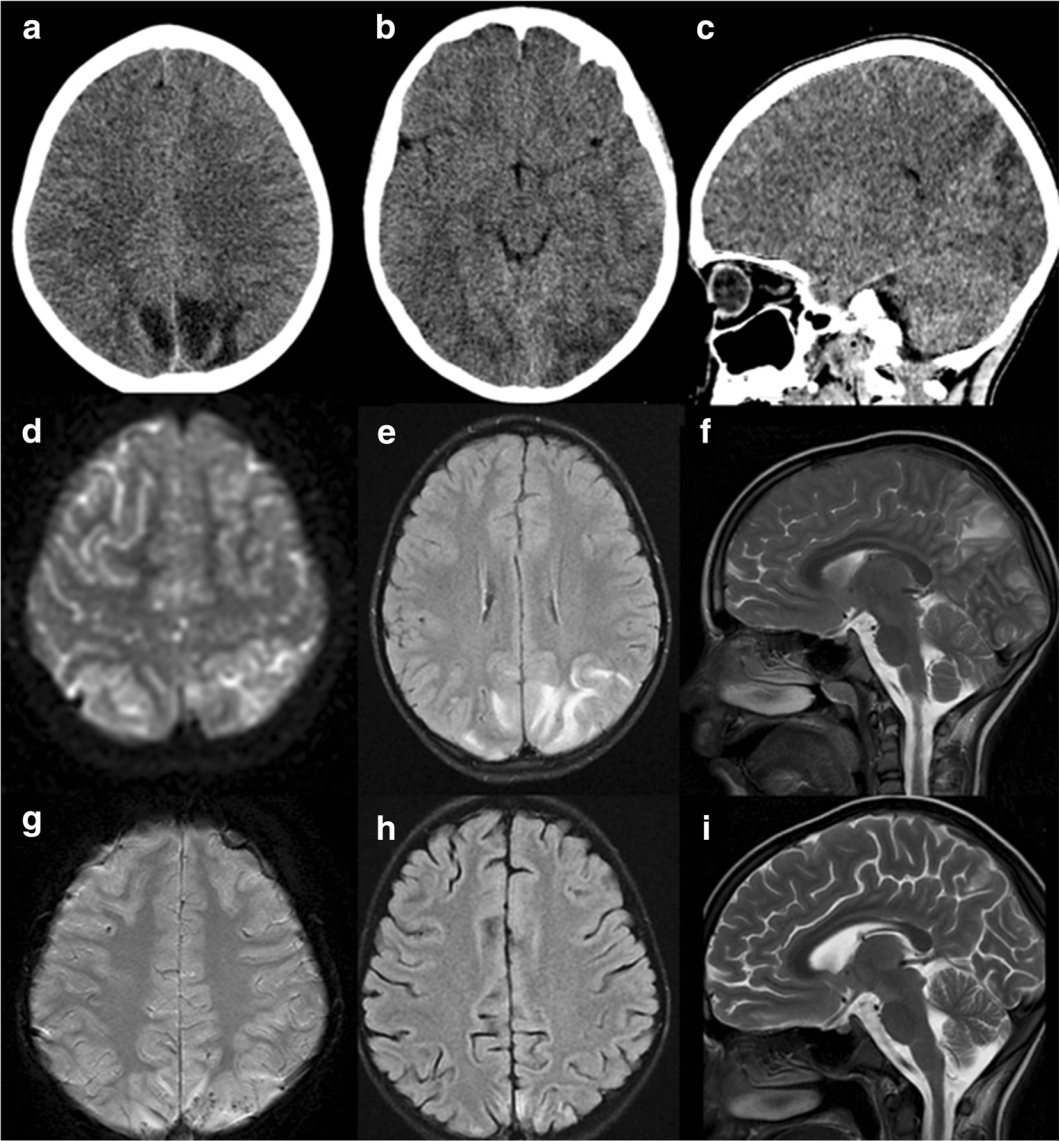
a–c Initial head CT axial plane (a and b) and sagittal multiplanar reconstruction (c): hypodense cortical-subcortical areas in the parietal lobes bilaterally and in the left occipital lobe—ischaemic changes with oedema. d–f Head magnetic resonance (MRI) in same day, axial diffusion-weighted image (DWI) (d): restriction of water diffusion, axial fluid-attenuated inversion recovery (FLAIR) image (e) and sagittal T2/weighted image (f): hyperintense signal in the parietal and occipital lobes—regions of cortical/subcortical oedema, correlating with PRES. g–i Control head MRI after 3 months, axial susceptibility weighted image (SWI) (g): small haemorrhagic regions, axial FLAIR image (h): substantial regression of changes in occipital lobes, sagittal T2/weighted image (i): small residual changes.
